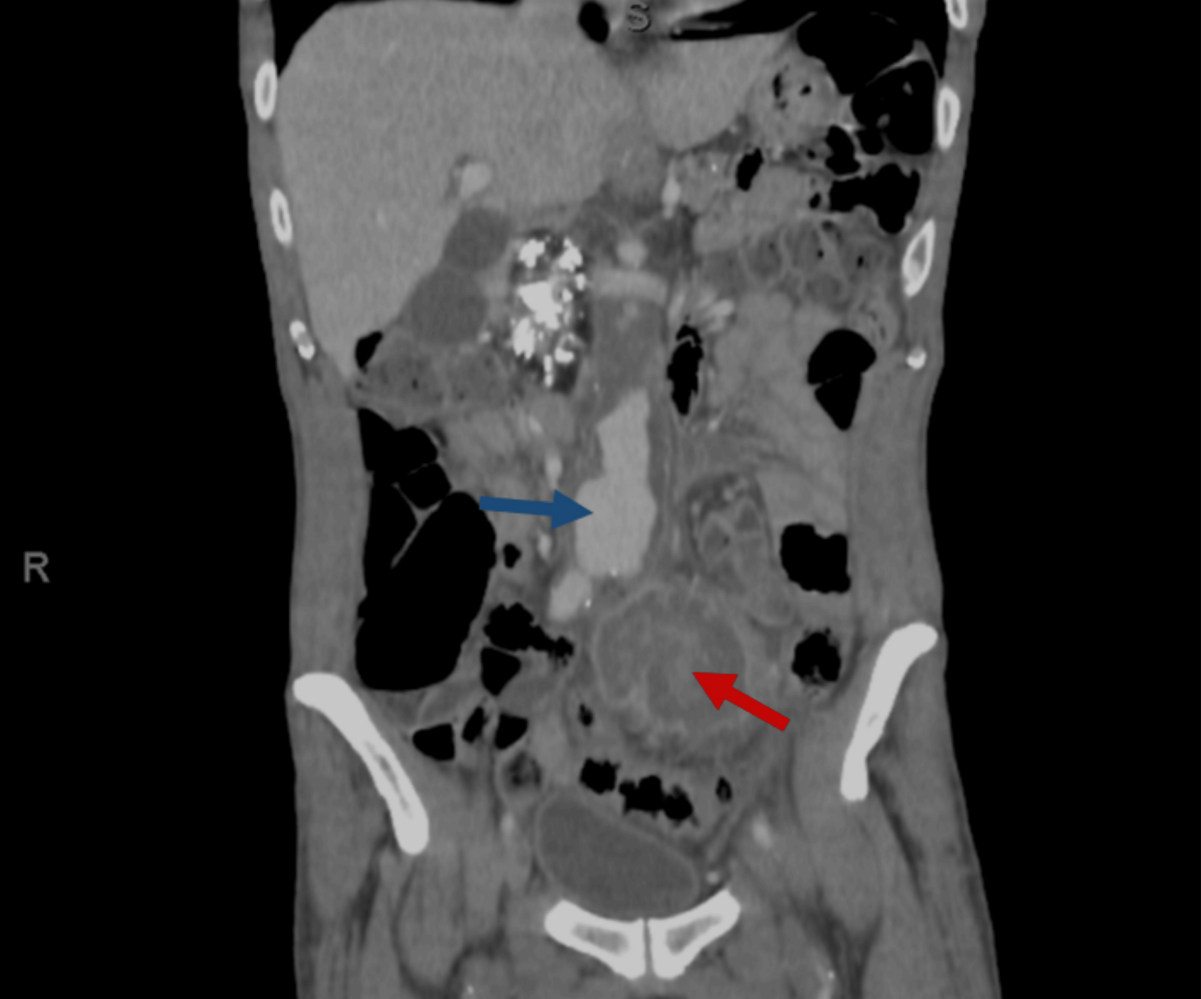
Coronal view of the computed tomography image. The red arrow indicates the left iliac artery pseudoaneurysm, and the blue arrow indicates abdominal aorta aneurysm
